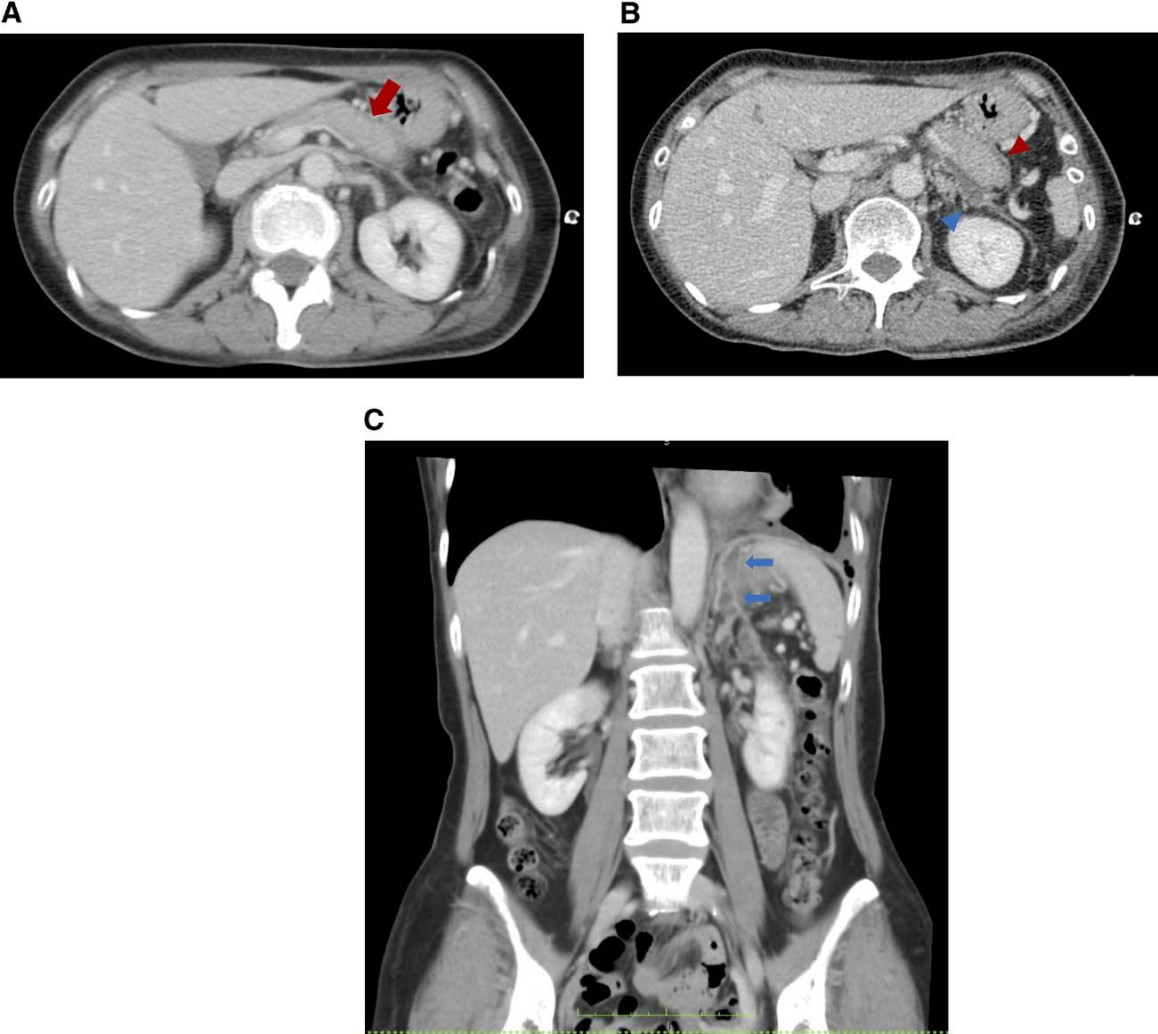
A, Thoracoabdominal contrast-enhanced CT showing diffuse enlargement of the pancreas (red arrow). B, A cyst with encapsulated fluid in the pancreatic tail (blue arrowhead) and a capsule-like rim around the pancreas (red arrowhead). C, Encapsulated fluid from the pancreatic tail to the left pleural cavity (blue arrows). CT, computed tomography.
